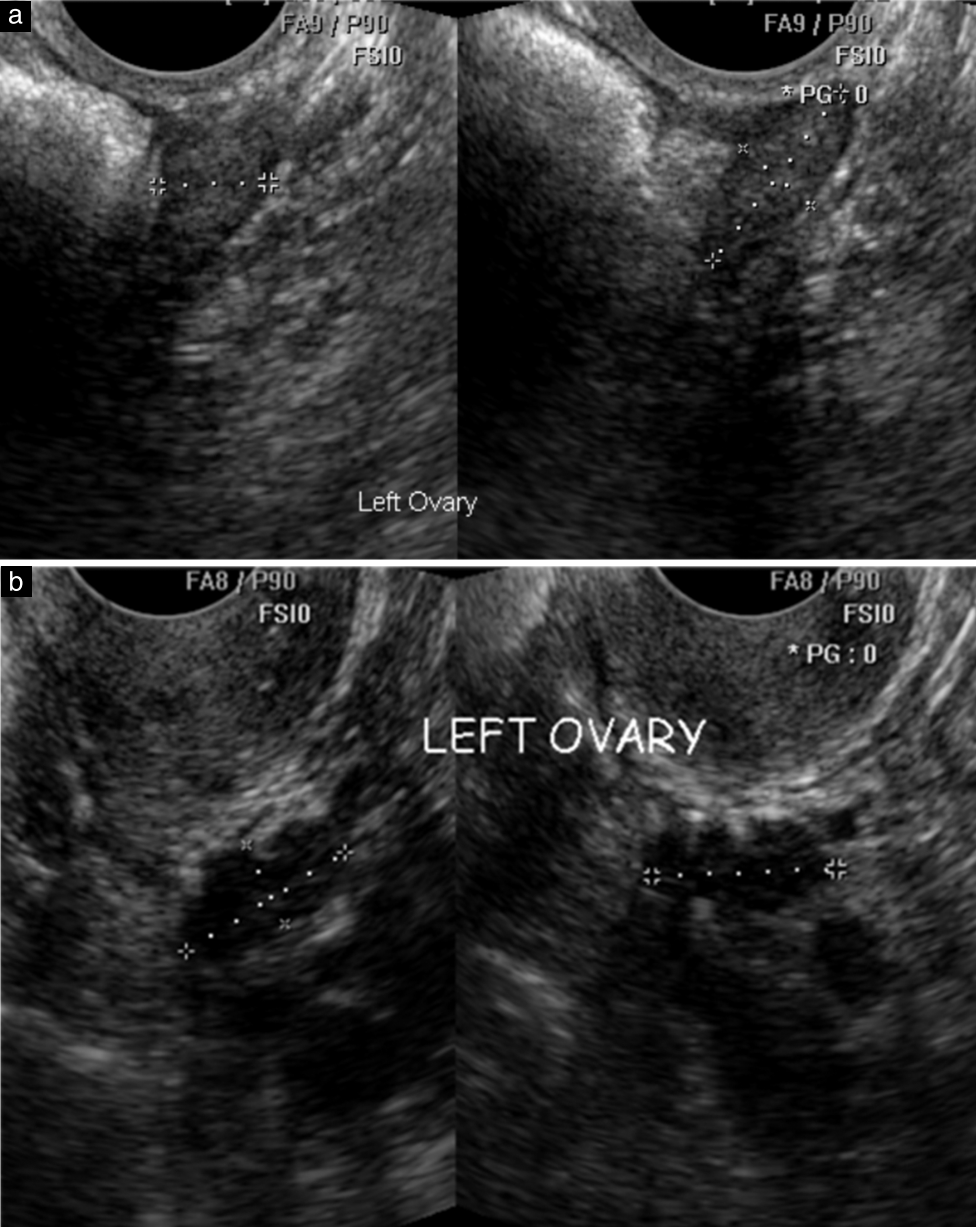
Longitudinal and transverse transvaginal ultrasound images acquired by sonographers to measure left ovaries in postmenopausal women. (a) The expert judged the ovary as normal and correctly measured by the sonographer. (b) The expert considered the sonographer had mistakenly measured a section of bowel rather than the ovary as the haustrations of large bowel are clearly visible in the structure marked by the calipers.
